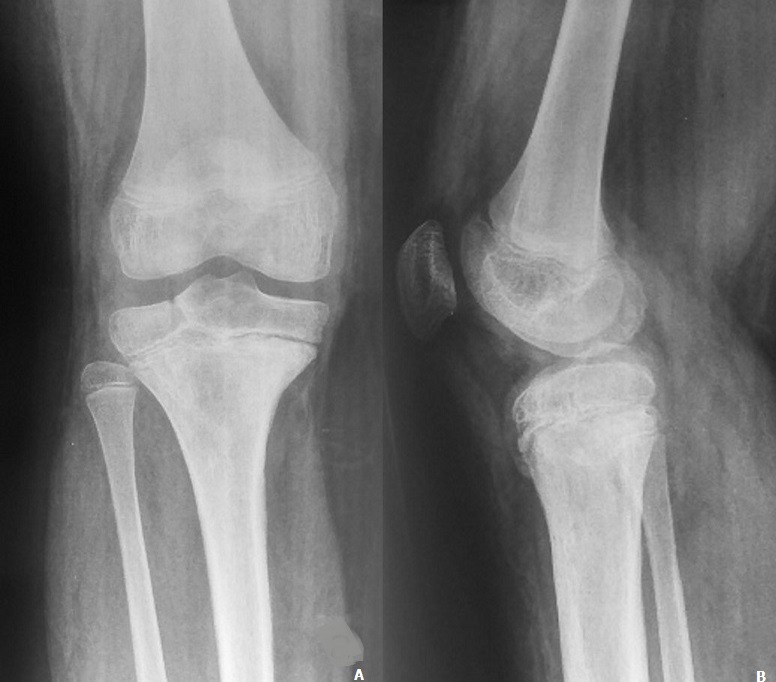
(A) anteroposterior and lateral (B) radiographs of the right knee with consolidation of the fracture at the last one year follow up
